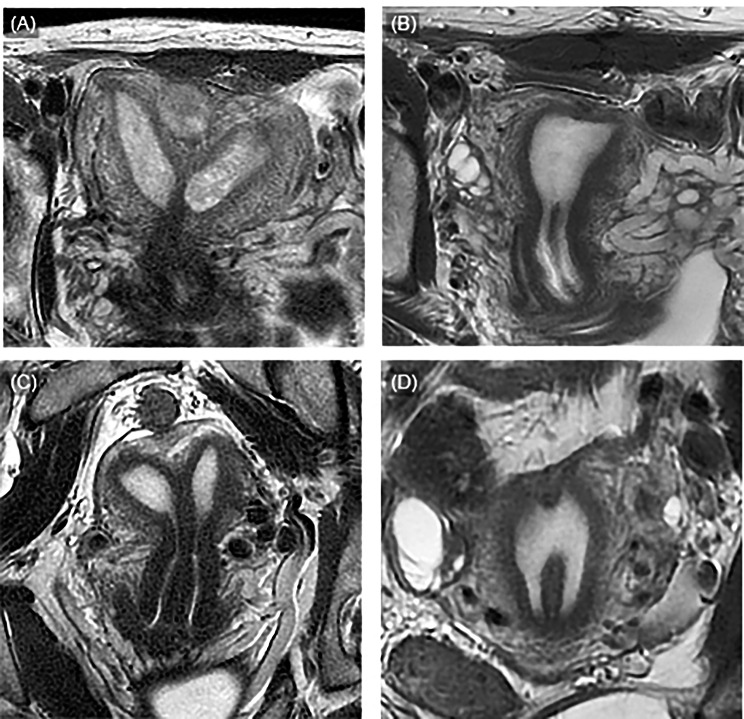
Imaging studies before and after ABM. Three cases are shown before and after ABM. (A) T2-weighed MRI of a septate uterus before metroplasty. The uterine shape was ESHRE/ESGE type U2a. (B) T2-weighed MRI after metroplasty for the case depicted in panel A. (C) T2-weighed MRI image before metroplasty. The uterine shape was ESHRE/ESGE type U2a. (D) T2-weighed MRI after metroplasty in the case depicted in panel C. MRI: magnetic resonance imaging, ESHRE: European Society of Human Reproduction and Embryology, ESGE: European Society for Gynaecological Endoscopy
